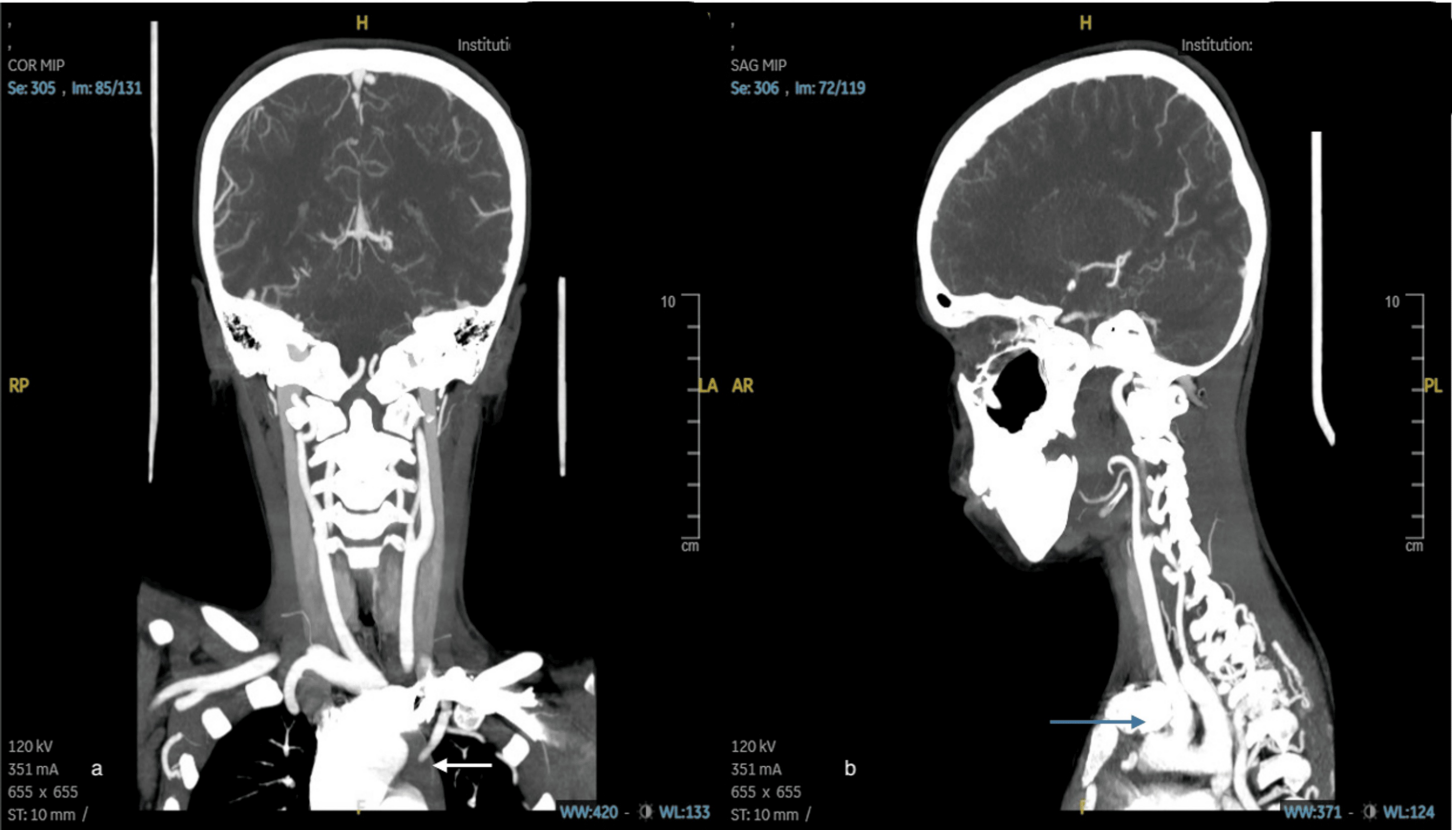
Computed tomography angiography of the carotid and aortic arch (a) coronal view (b) sagittal view showing narrowing of the posterior aortic arch with a focal low severe stenosis at the junction of the aortic arch and descending aorta. The caliber of the partially imaged the descending aorta is significantly reduced, and multiple collaterals are observed along the cervical thoracic posterior paraspinal space.
